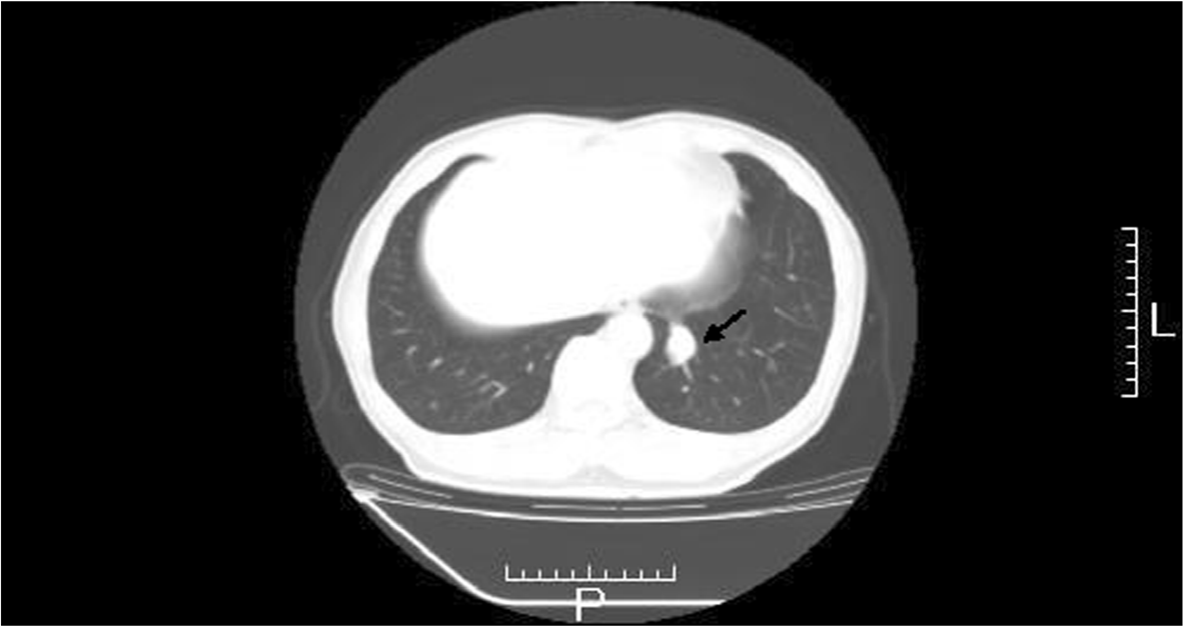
Chest computed tomography on 21 May 2008. Chest computed tomography shows a well-demarcated 20-mm mass at S10 of the left lung (*arrow*)
